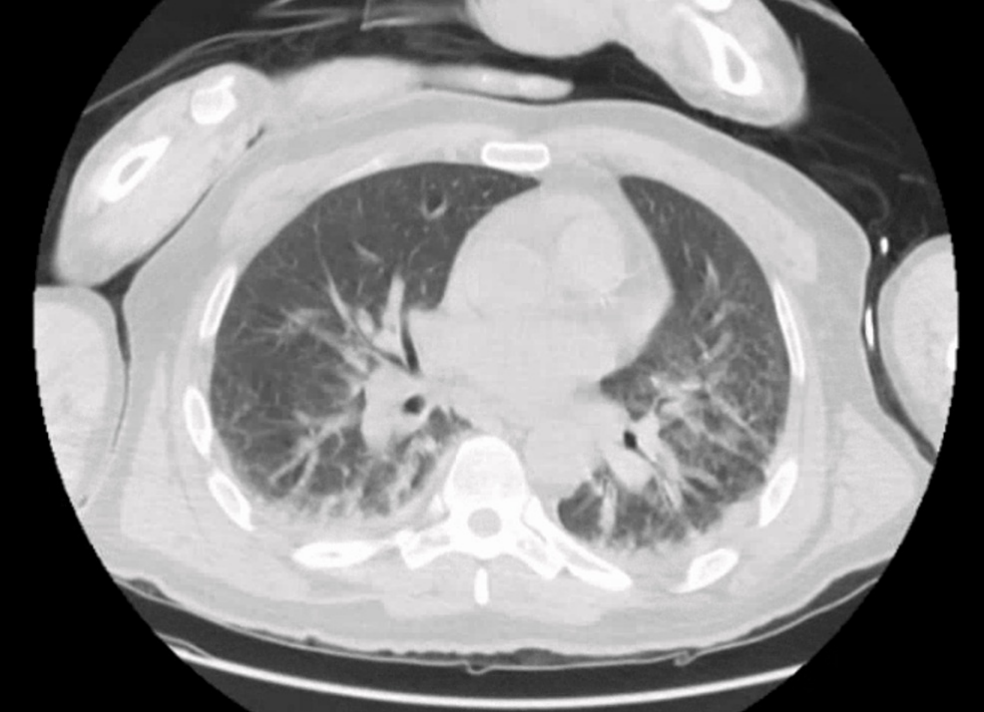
Chest CT showing bilateral bibasilar consolidations consistent with pneumonia and bilateral trace pleural effusion
